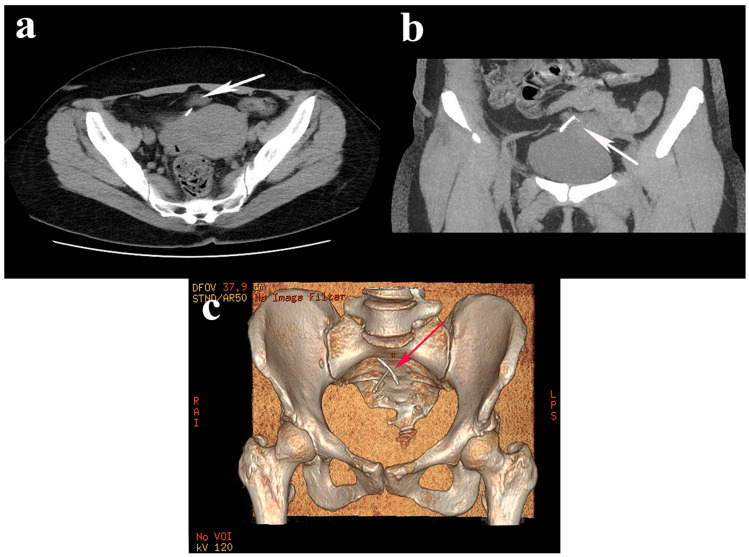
Axial (a) and sagittal (b) abdominopelvic computed tomography images revealed a translocated IUD above the bladder and uterus (white arrows), oriented slightly obliquely to the right; (c) 3D reconstruction of the IUD topography (red arrow).
